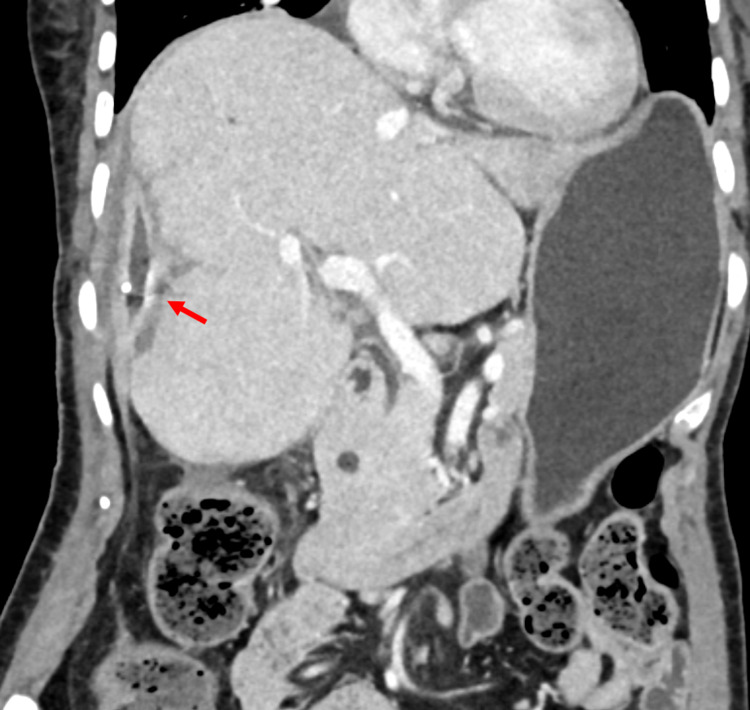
Contrast-enhanced CT performed one month after the ERCP and 20 days after catheter drainage showing almost complete disappearance of perihepatic fluid collection (arrow)
